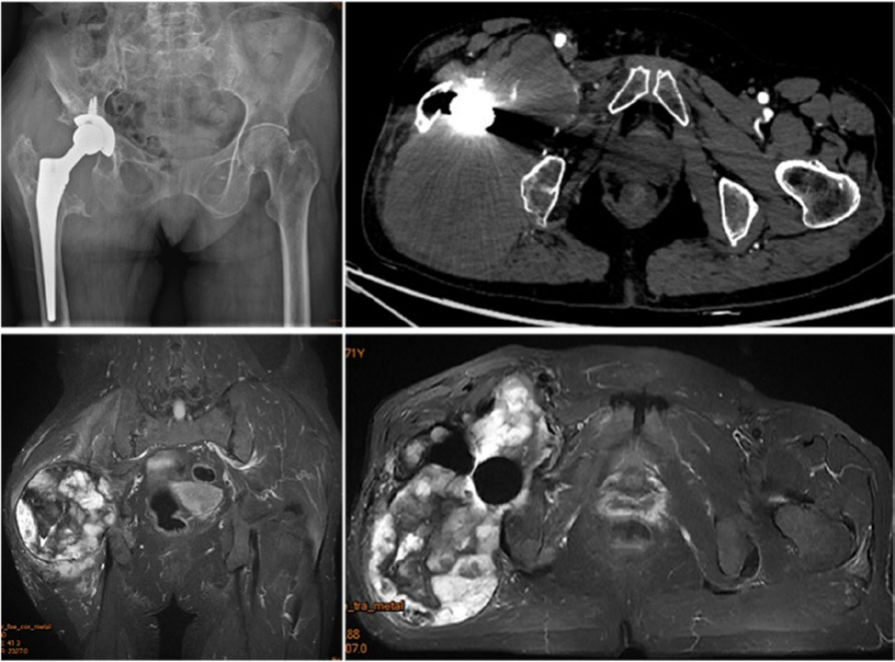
One CEH case: x-ray, CT, and MRI 20 years after primary THA
